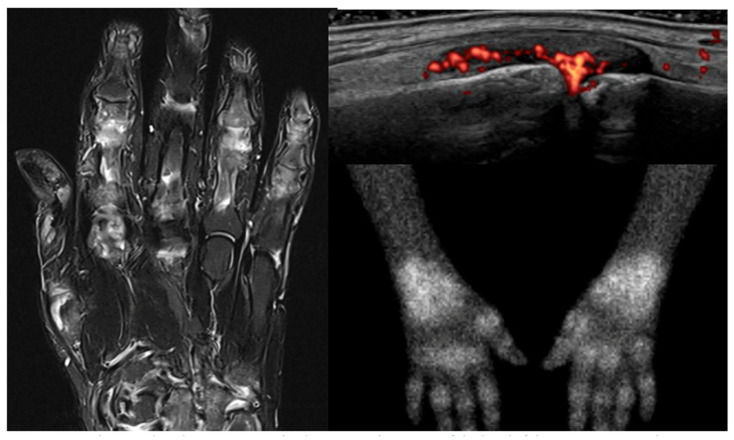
MRI, ultrasound, and 99mTc-Maraciclatide scintigraphy images of the hand(s) of the same patient with rheumatoid arthritis.
